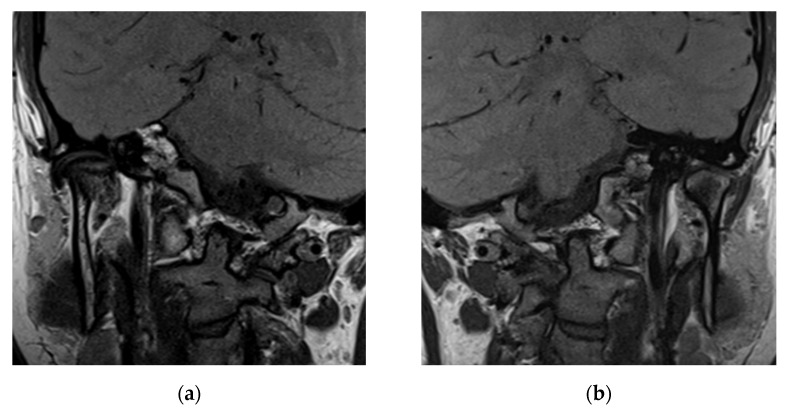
Coronal proton density MRI of the temporomandibular joints: (a) left and (b) right lateral (external) disk displacement with modified condyle shape.
